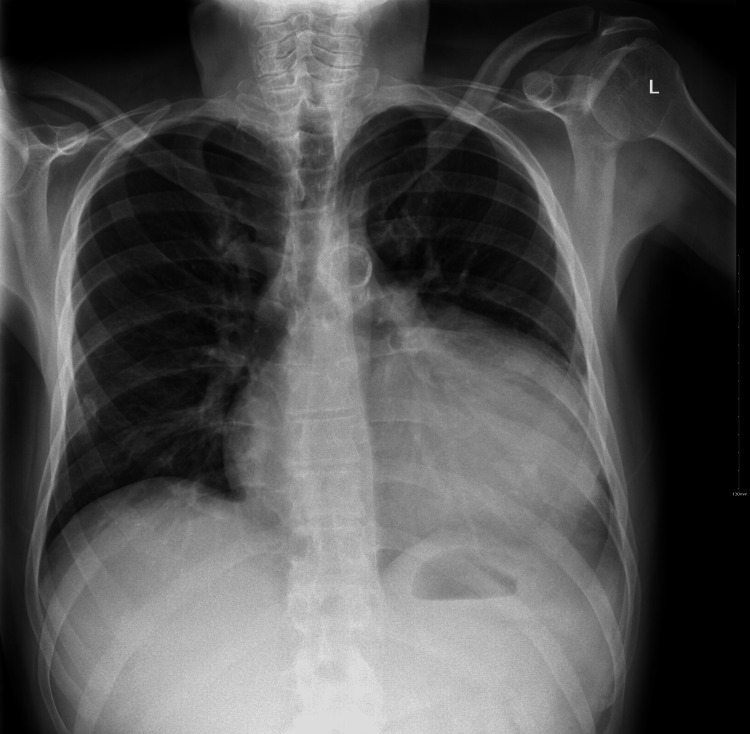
Chest X-ray depicting enlargement of the cardiac silhouette.
